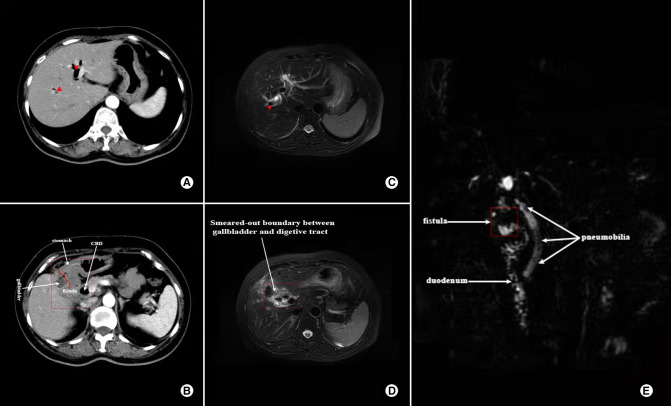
Contrast-enhanced CT and MRCP images showing the characteristics of a 57-year-old female who was diagnosed with both CDF and CGF; (A) CT image of Pneumobilia (red arrow); (B) CT image showing ill-defined borderline between gallbladder and digestive tract (marked in the red box); (C) MRCP image showing Pneumobilia (red arrow); (D) MRCP image of ill-defined borderline between gallbladder and digestive tract (marked in the red box); (E) Reconstruction of MRCP showing Pneumobilia and fistula (white arrow).
